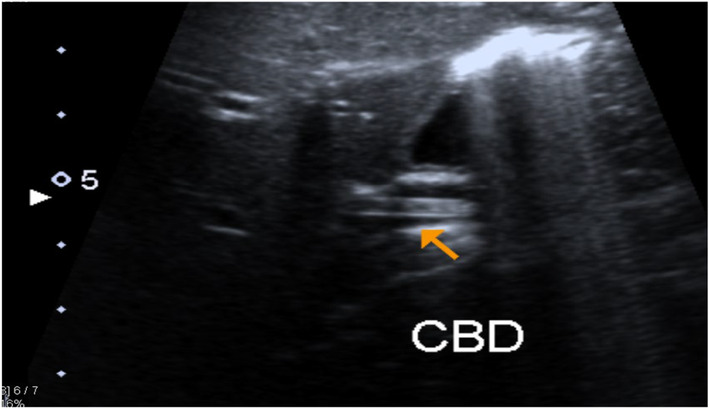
Hepatobiliary ultrasound of the patient.
